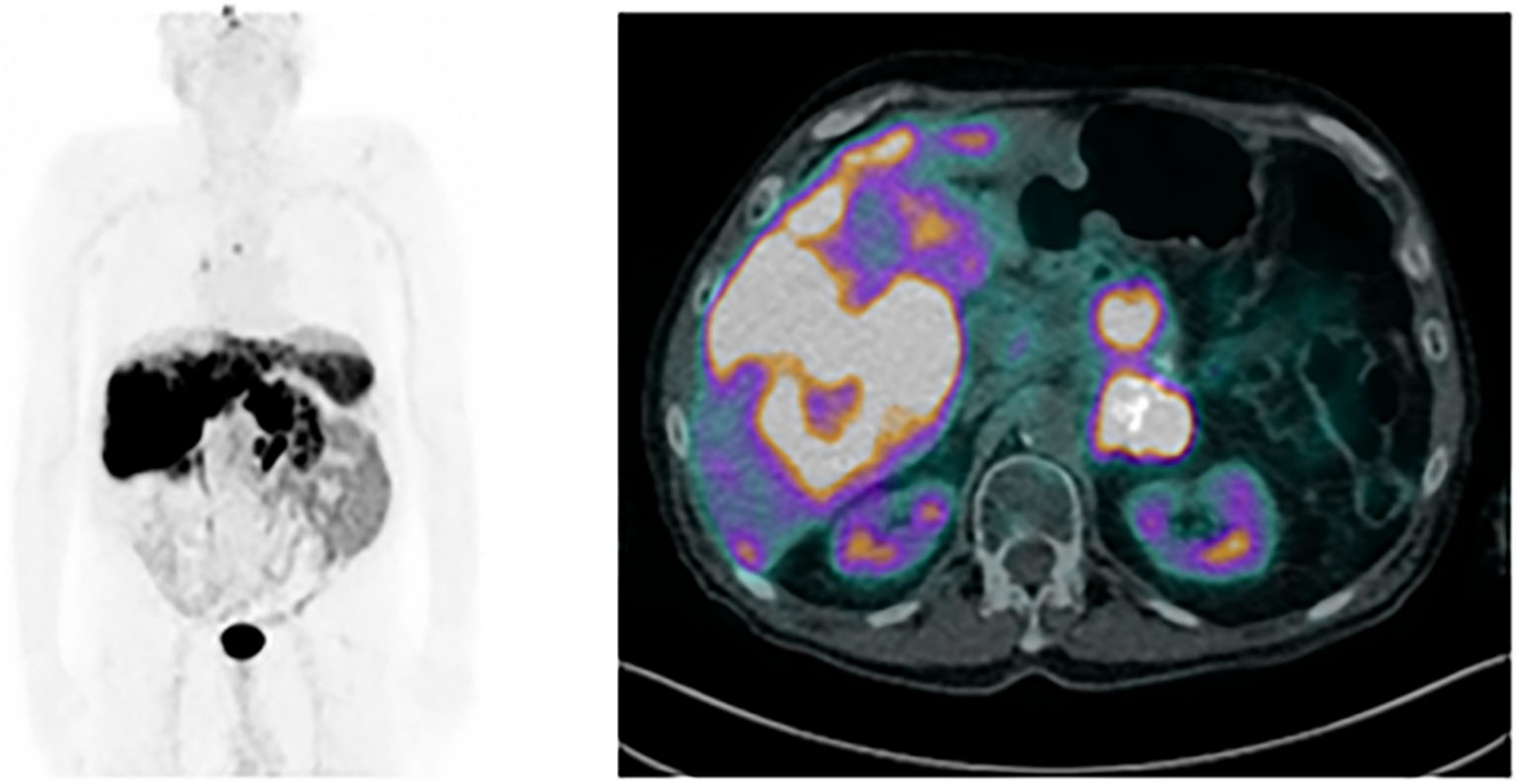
[^68^Ga]Ga-DOTA-NOC PET/CT scan performed at the time of insulinoma diagnosis; on the left, the maximum intensity projection shows uptake in several hepatic lesions and discrete uptake in lymph node lesions, including the left thoracic para-aortic, interaortocaval, and left lumboaortic chains, and right perihilar region, compatible with metastatic disease; on the right, the axial fused PET/CT image shows heterogeneous uptake in several hepatic lesions, compatible with metastases.
